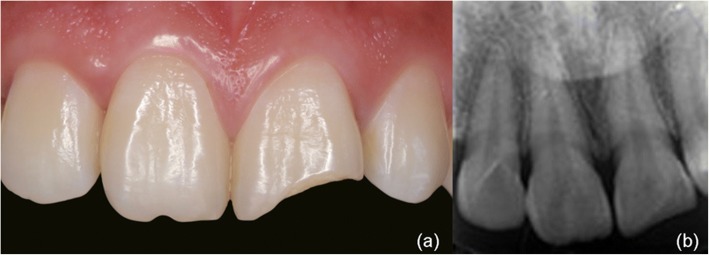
(a) Preoperative view of fractured maxillary anterior teeth. (b) Initial radiographic view of anterior teeth
